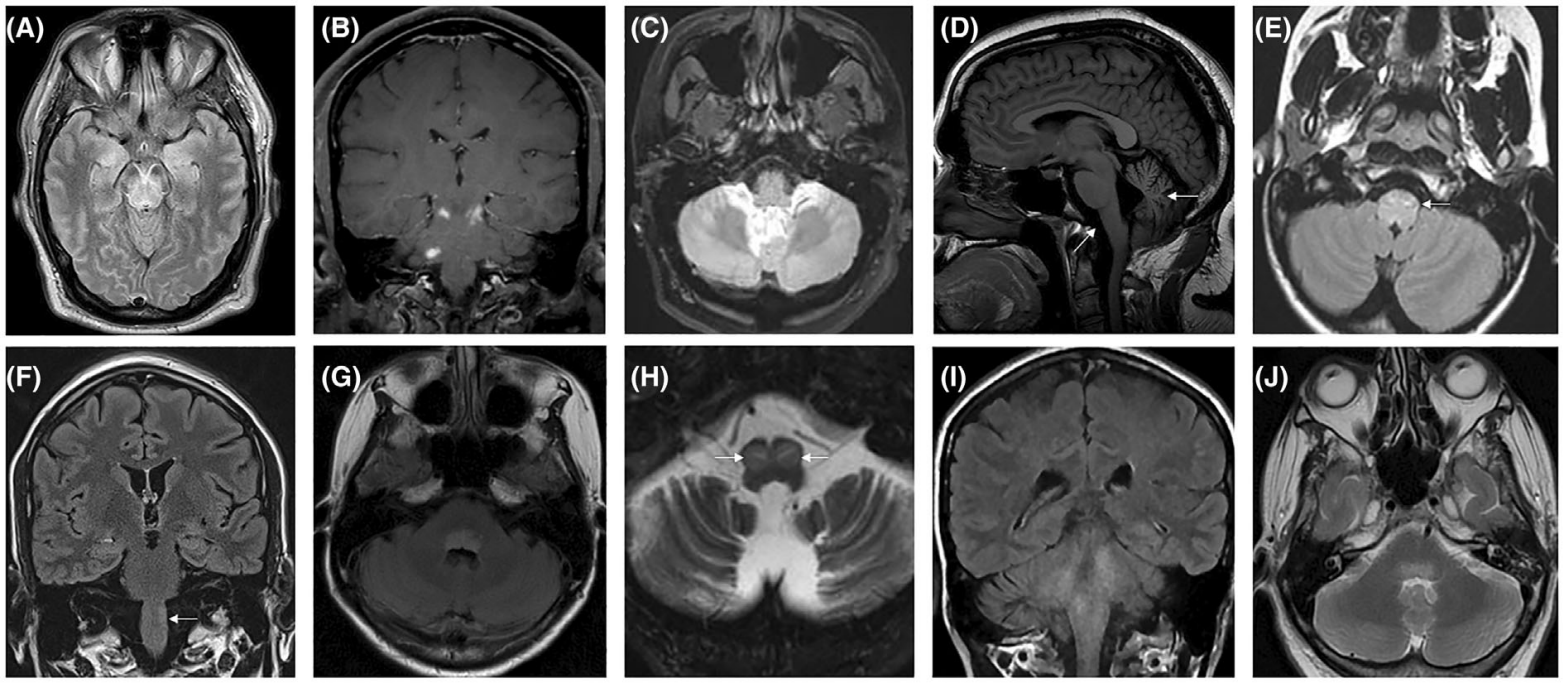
Selection of MRI abnormalities from patients with autoimmune brainstem encephalitis. (A) Axial T2 FLAIR image demonstrating midbrain signal change and bilateral mesial temporal lobe signal changes in a 46‐year‐old male with subacute onset ptosis, ophthalmoplegia and ataxia, positive for Ma2‐IgG. (B) Coronal gadolinium‐enhancing lesions affecting brainstem and cerebellum in 27‐year‐old female with MOGAD. (C) Axial T2 FLAIR signal change of cerebellum in a 50‐year‐old male with sensorineural hearing loss, diplopia, vertigo, and cerebellar ataxia and positive for KLHL11‐IgG. (D) Sagittal T2 image demonstrating severe brainstem and cerebellar atrophy (arrows) in a 20‐year‐old male with diplopia and ataxia, positive for ITPR‐1‐IgG. (E) Axial T2 FLAIR signal change involving the medulla in 10‐year‐old boy with GAD65‐IgG‐associated brainstem encephalitis. (F) Coronal T2image demonstrating signal change in left medulla (arrow) of 28‐year‐old male with autoimmune brainstem encephalitis associated with Ma2‐IgG and embryonal cell carcinoma of testis. (G) Axial T2 FLAIR image demonstrating signal change in dorsal pons in a 53‐year‐old female with AQP4 + NMOSD. (H) Axial T2 signal change in the medullary olives consistent with bilateral hypertrophic olivary degeneration in a 44‐year‐old male with palatal myoclonus, positive for KLHL‐11 IgG. (I) Coronal T2 FLAIR signal change affecting medulla, pons and cerebellum in 48‐year‐old woman with autoimmune brainstem encephalitis associated with NMDA‐R, GAD65 and GQ1B‐IgGs. (J) Axial T2 signal change in the dorsal pons in 45‐year‐old male with KLHL‐11‐IgG‐associated rhombencephalitis and germ cell tumor of testis.
